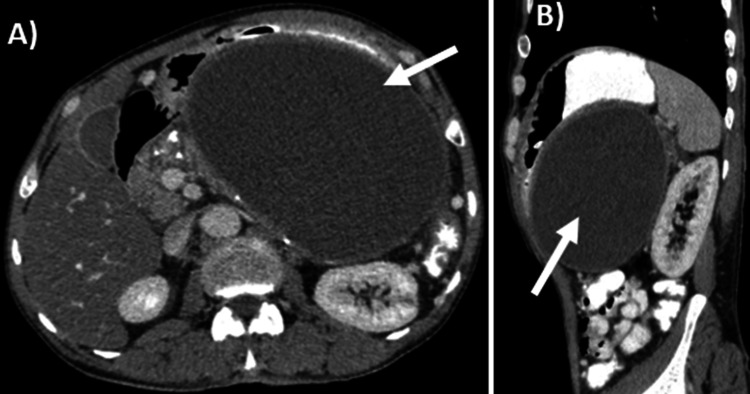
CECT: abdomen and pelvis (axial and sagittal cuts) demonstrating a predominantly cystic lesion compressing the stomach and left lobe of the liver A) Axial cut: CECT (Abdomen): Portal venous phase A large, well-defined, hypodense, uniformly cystic lesion of size 136*117*122 mm (white arrow) is seen arising from the body of the pancreas, causing severe compression of the gastric antrum. It is seen extending in the gastrohepatic ligament between the stomach and left hepatic lobe, showing a peripheral solid component. The pancreas appeared bulky, with no per-pancreatic fat stranding. No obvious communication was noted with the main pancreatic duct (MPD). B) Sagittal cut: CECT (Abdomen): Portal venous phase A fairly large cystic, hypodense lesion occupying the lesser sac was seen abutting and causing compression of the gastric antrum. It showed peripheral enhancement in relation to the left lobe of the liver, indicating a solid component; however, with preserved fat planes. There is no associated lymphadenopathy. Findings were suggestive of a pseudocyst of the pancreas or gastric GIST. CECT: Contrast-enhanced computed tomography
